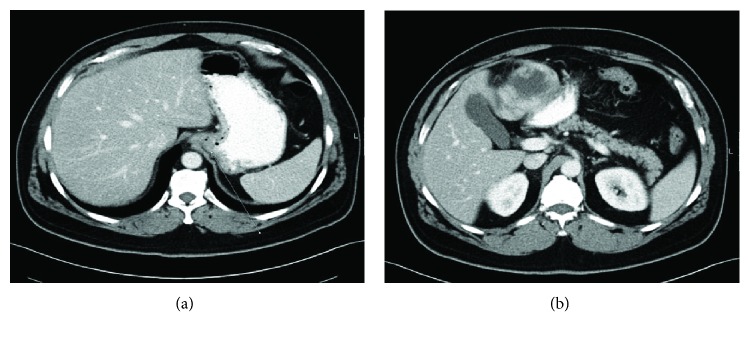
Infused CT of the abdomen. (a) Circumferential thickening of the lower esophagus. (b) A large mass with peripheral enhancement and central necrosis most likely representing gastric GIST.
